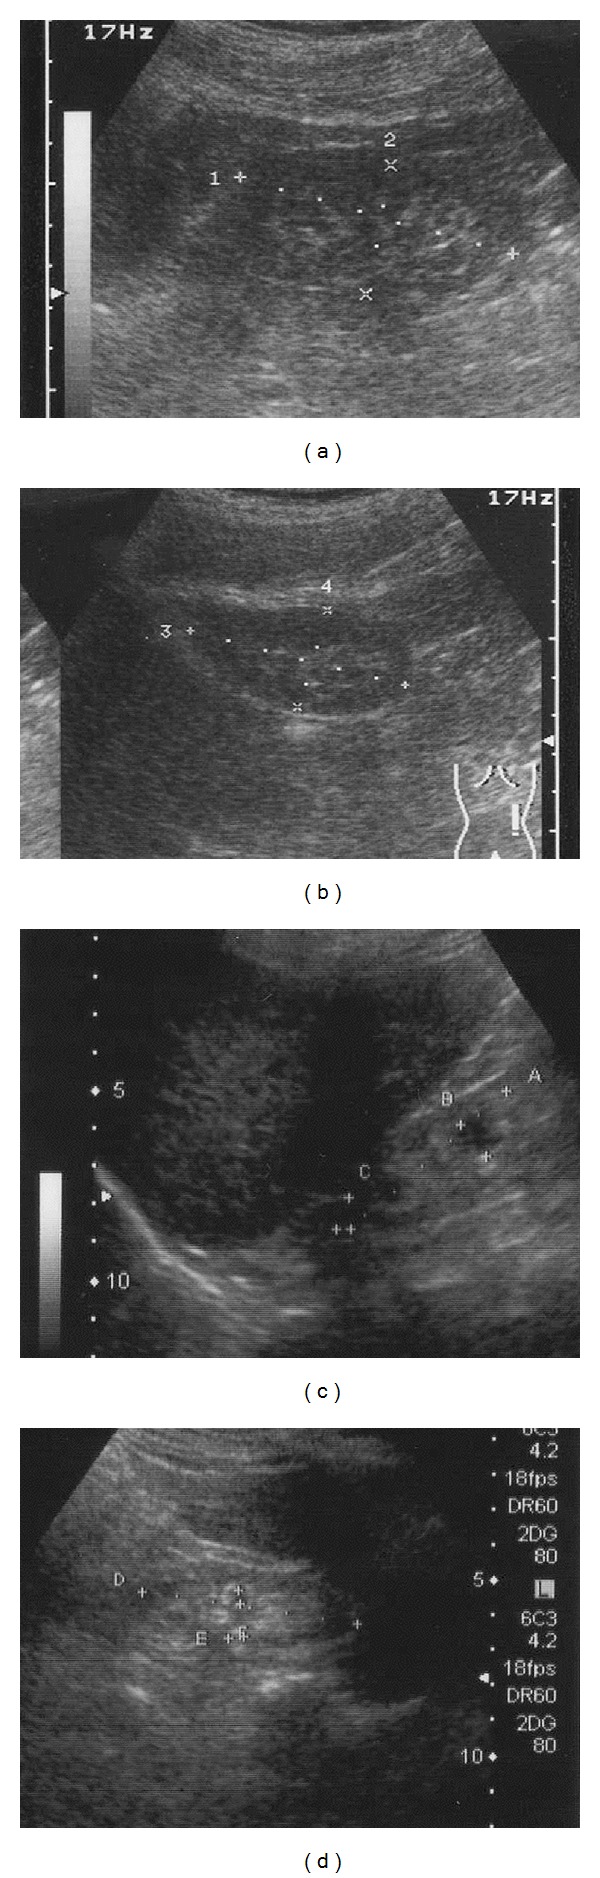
Example of sonographic measurements of the same patient. First measurement (a) right, (b) left, 4 years 9 months after the first measurement (c) right, (d) left.
